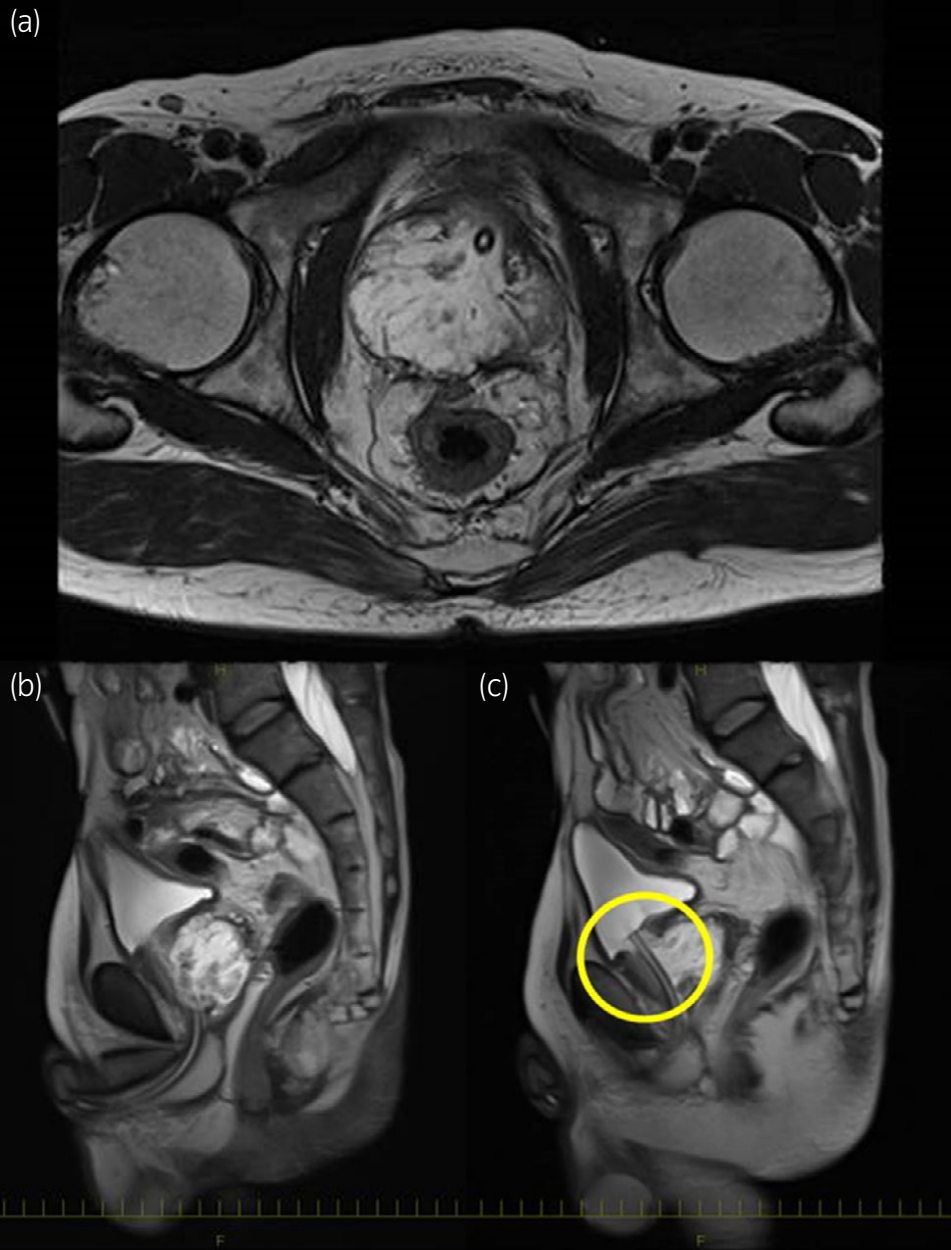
MRI of the pelvis at diagnosis. (a,b) Transverse and sagittal sections of T2‐weighted MRI: A mucus‐enriched prostate tumor was detected, similar to the contrast‐enhanced CT and PET‐CT images. (c) Sagittal section of T2‐weighted MRI suggesting that a mucinous tumor of the prostate extended to the muscle of the bladder (inner yellow circle).
